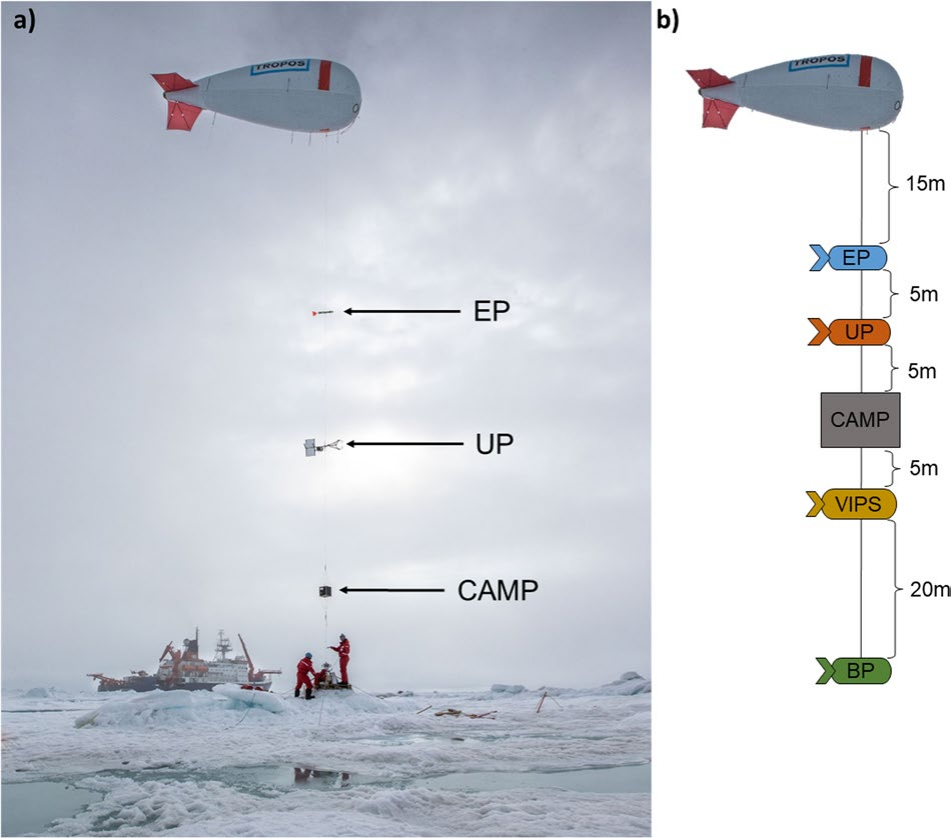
(a) Instruments attached to the tether of the balloon: extended meteorology package (EP), ultrasonic anemometer package (UP), and cubic aerosol measurement platform (CAMP). Photo credit: Lianna Nixon/CIRES at University of Colorado, Boulder. (b) Sketch of the typical instrument arrangement and dimensions; not all instruments were operated simultaneously.
